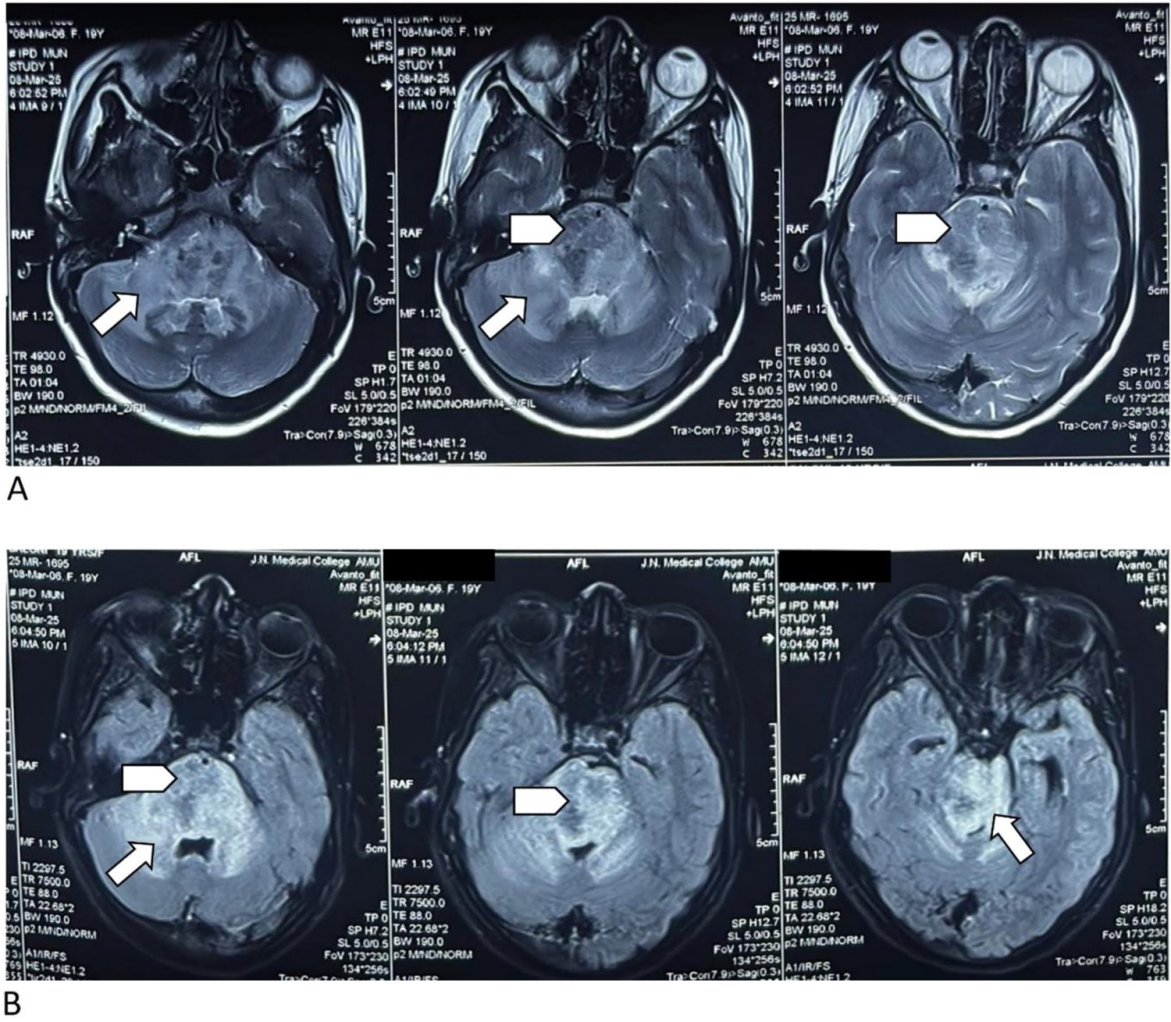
T2-weighted and FLAIR axial MRI brain sequences showing brainstem tuberculoma with surrounding edema (arrows and arrowheads). (A) Axial T2-weighted images showing a well-defined, conglomerated hypointense lesion in the right midbrain and pons (arrowhead), with marked perilesional hyperintensity consistent with vasogenic edema (arrows). The lesion causes mass effect with distortion of the fourth ventricle and mild effacement of perimesencephalic cisterns. (B) Corresponding FLAIR axial images showing the lesion (arrowhead) as heterogeneous hyperintensity, with marked perilesional edema (arrows) extending along the brainstem and posterior fossa.
